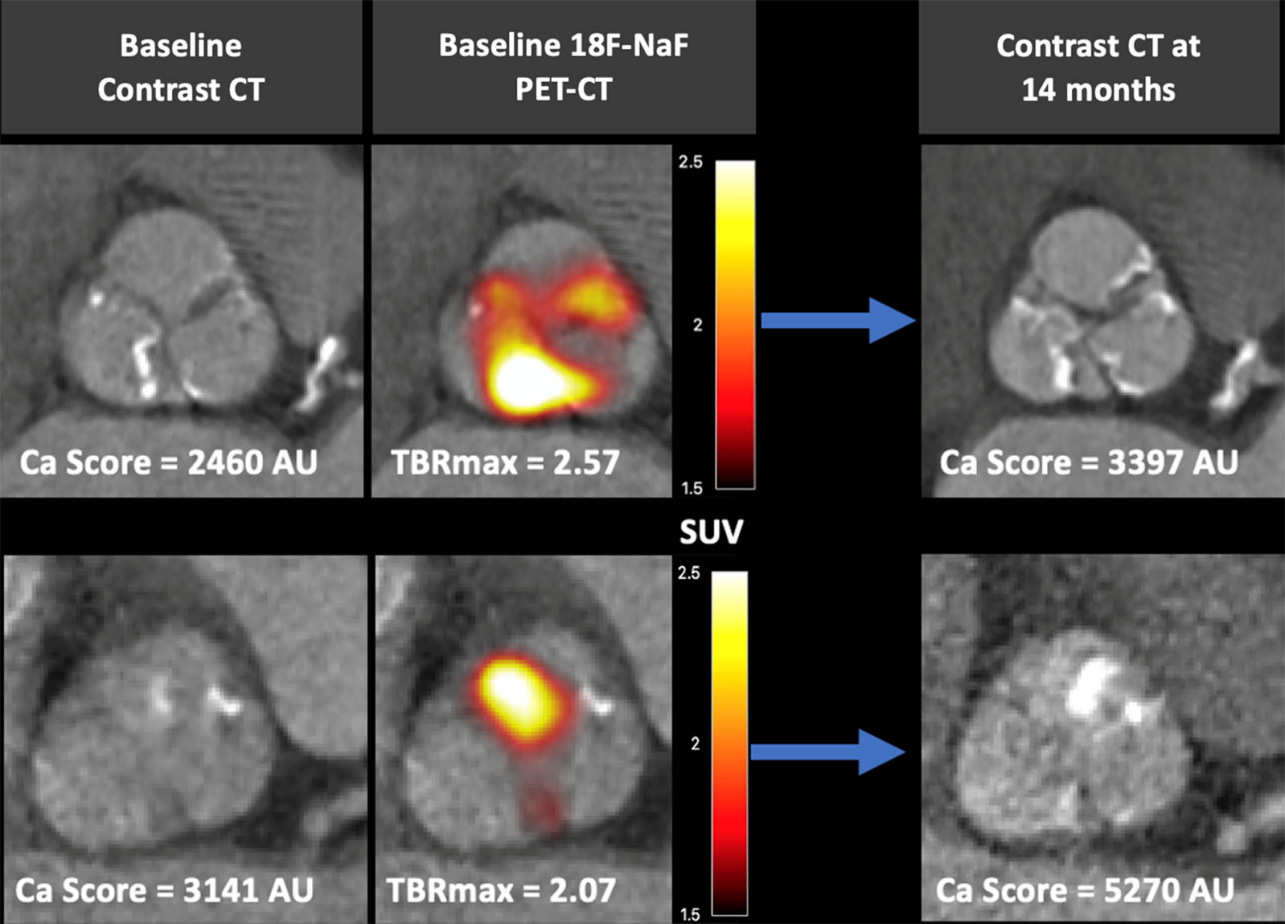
Two patients with progressive calcific aortic valve disease. (Left) Baseline computed tomography (CT) images. (Middle) Fused positron emission tomography (PET)-CT images showing increased ^18^F-fluoride valvular uptake (red/yellow areas). (Right) Repeat CT scans after 14 months with progressive aortic valve calcium score and new macroscopic calcium (white areas) in a similar distribution to that of baseline PET uptake. *18F-NaF PET-CT*, ^18^F-sodium fluoride; *PET*, positron emission tomography; *CT*, computed tomography; *Ca Score*, Calcium score in Agatston units; *TBRmax*, maximum tissue-to-background ratio
